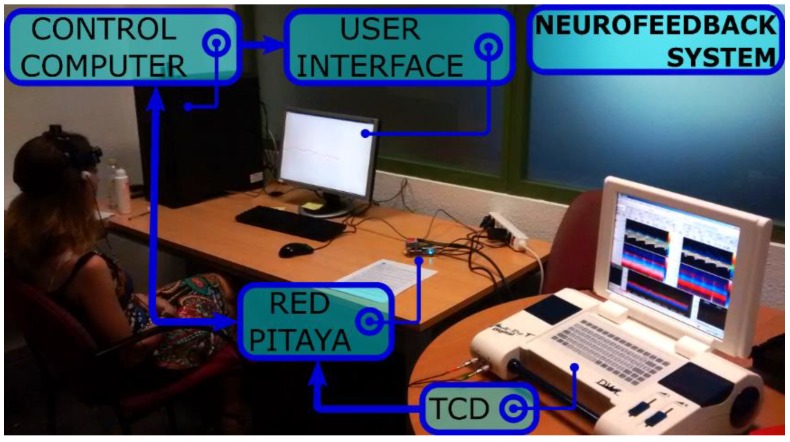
Different parts of the developed TCD-based neurofeedback system can be observed in the figure. The TCD ultrasound device registers the cerebral blood flow velocity (CBFV) signals from the participant. The analog output from this device is connected to the Red Pitaya board, which acquires the signals and delivers them to the control computer by Ethernet. The control computer receives the signals and executes the neurofeedback application. The feedback is provided to the participant through the computer screen (user interface).
